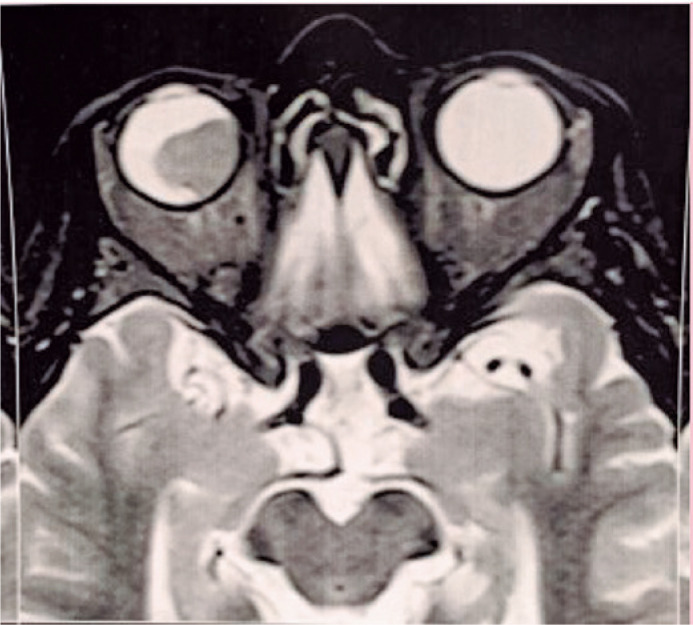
Magnetic resonance imaging showing intraocular mass.
